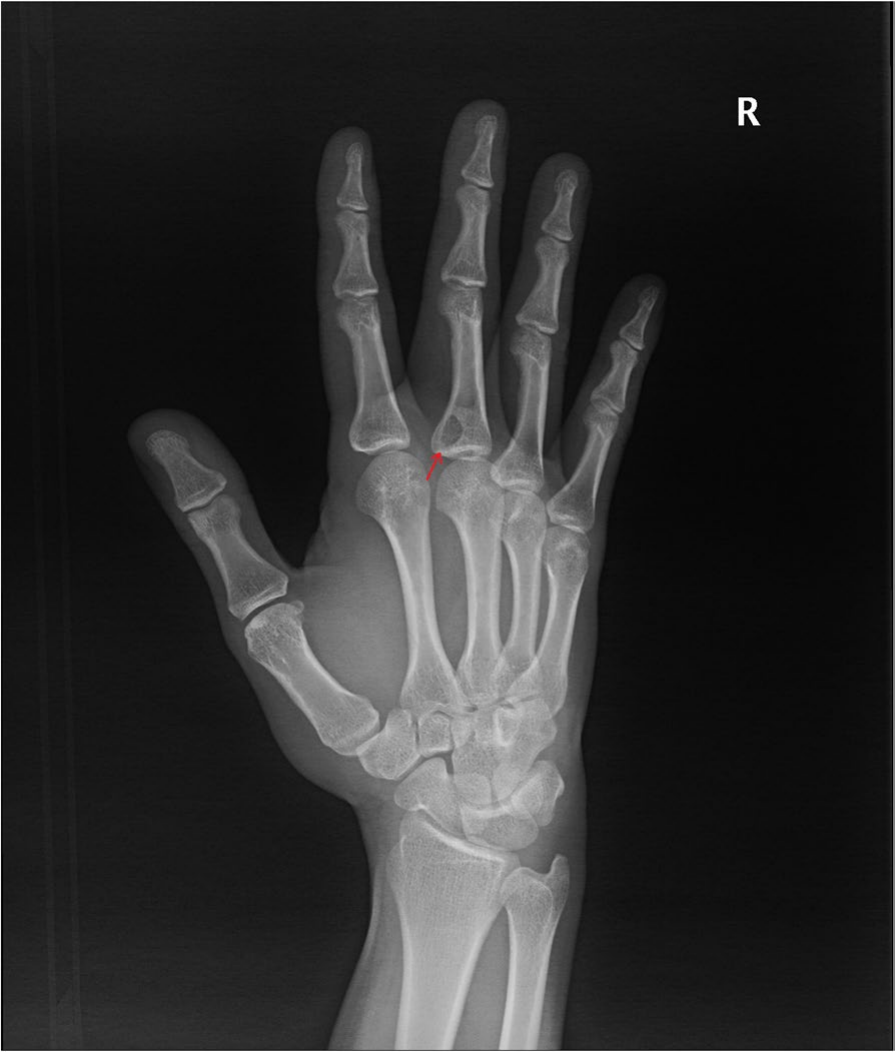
X-ray revealed a bony defect of right third proximal phalanx (red arrow)
